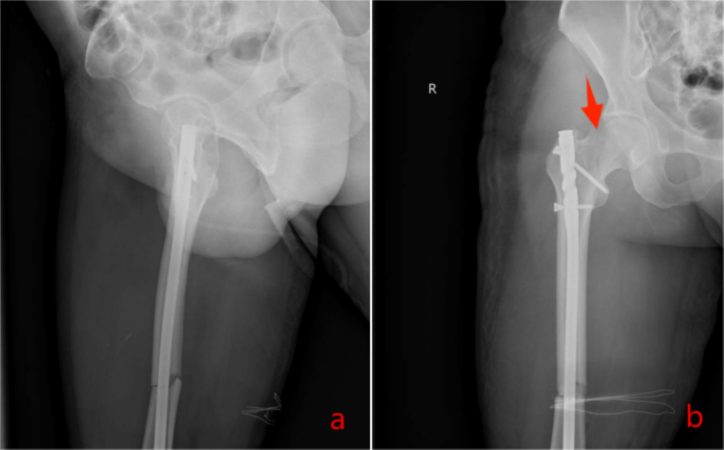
Postoperative day 2 X-ray showed a basicervical femoral neck fracture (Pauwels type III).
